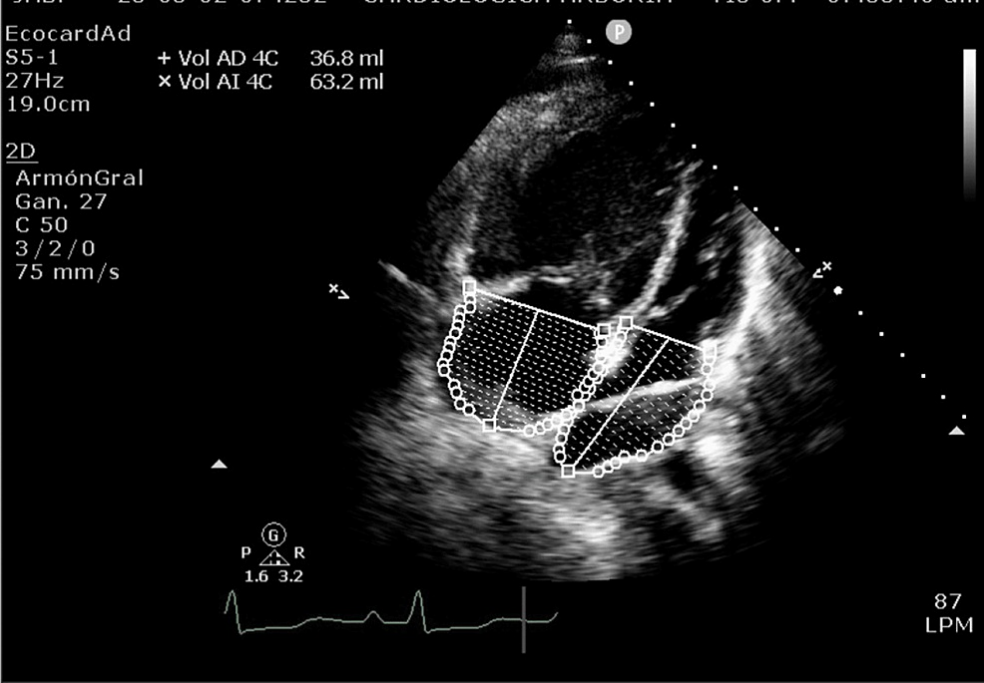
Echocardiogram of four chambers, visualizes left restrictive cor triatriatum The left restrictive cor triatriatum. Situs solitus, atrial and visceral, in levocardia, with concordant atrioventricular and ventriculoarterial connections in the perforated mode. No evidence of intracardiac shunts. Normal pulmonary and systemic venous connections
